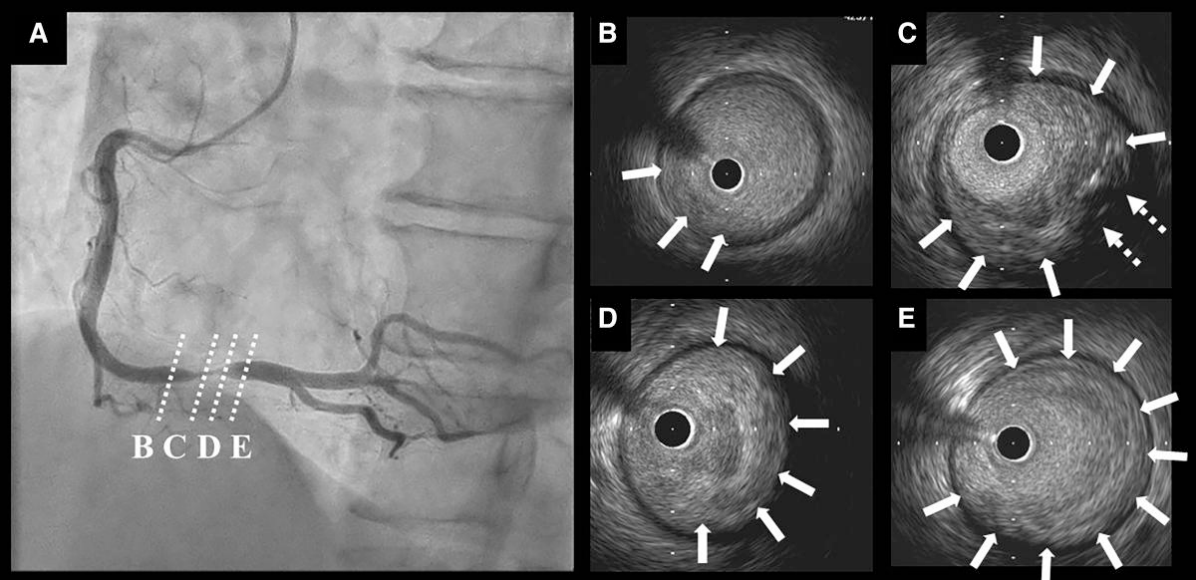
The coronary angiogram of the right coronary artery in the left anterior oblique view (*B*). The intravascular ultrasound (IVUS) images (*B–E*) reveal a significant stenosis (*C, D*), which corresponds to the site of the cavo-tricuspid isthmus ablation. Intravascular ultrasound also demonstrated that this stenotic lesion primarily consisted of heterogeneous fibrous plaque (solid arrows) including few echolucent lesions (dashed arrows).
